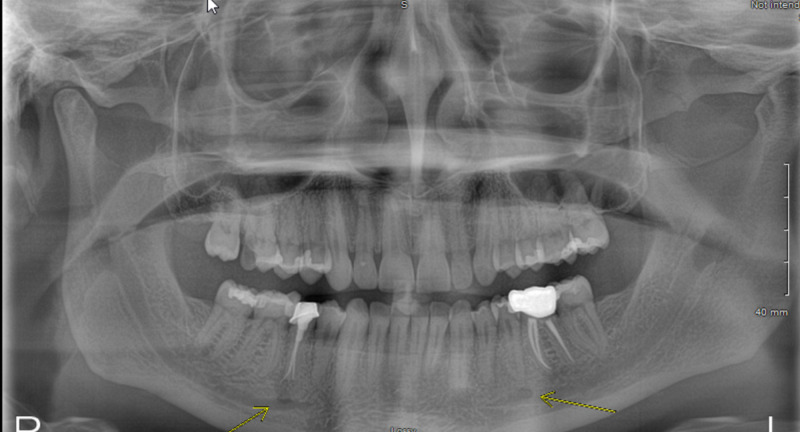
X-ray panorex orthopantogram showing dental abscesses in teeth #20 and #29 (see arrows)
